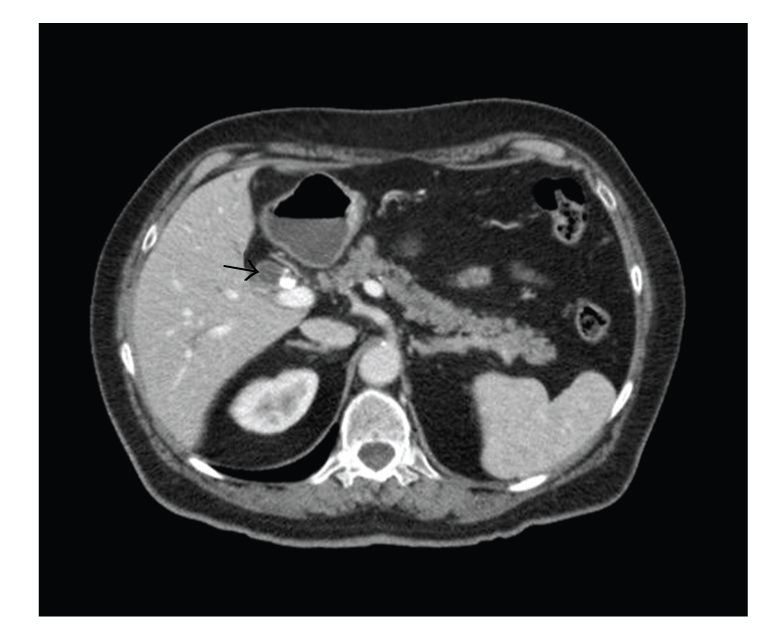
CT demonstrating lesion arising from common bile duct (arrow) with the common bile duct stent seen more medially.
